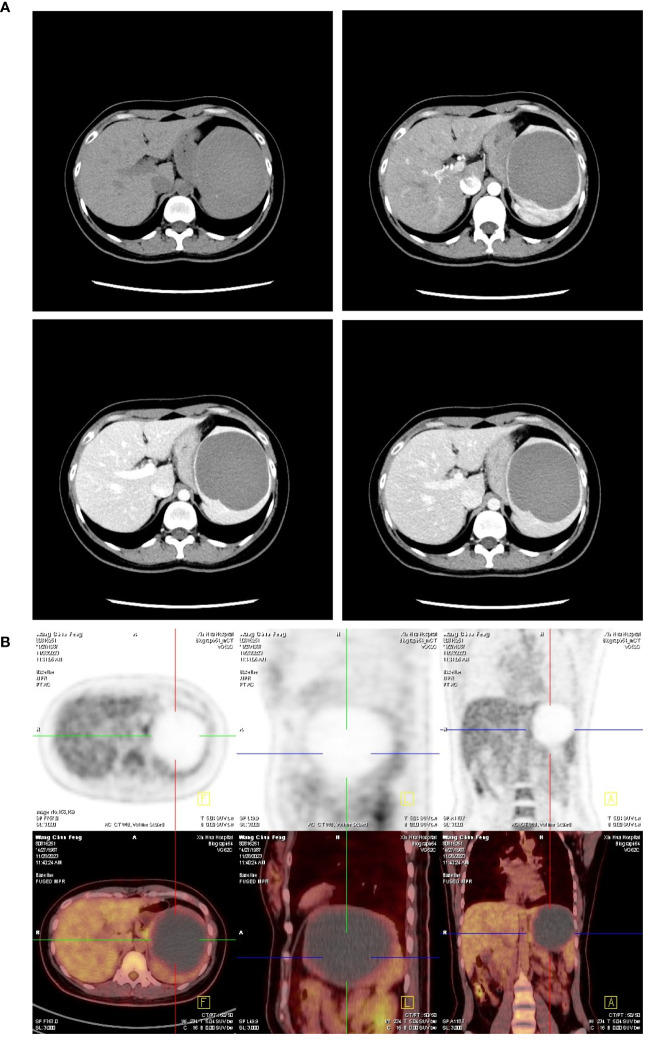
Abdominal imaging of the splenic cyst before surgery. (A) Representative four-phase image (non-contrast, arterial, portal, and liver phase) of the splenic cyst on contrast-enhanced CT scan showed no nodule or disproportionate thickening on the cystic wall except for tiny calcification was observed. There was also no contrast enhancement for the whole lesion constantly. (B) PET/CT scan showed no 18F-FDG uptake by the splenic lesion.
